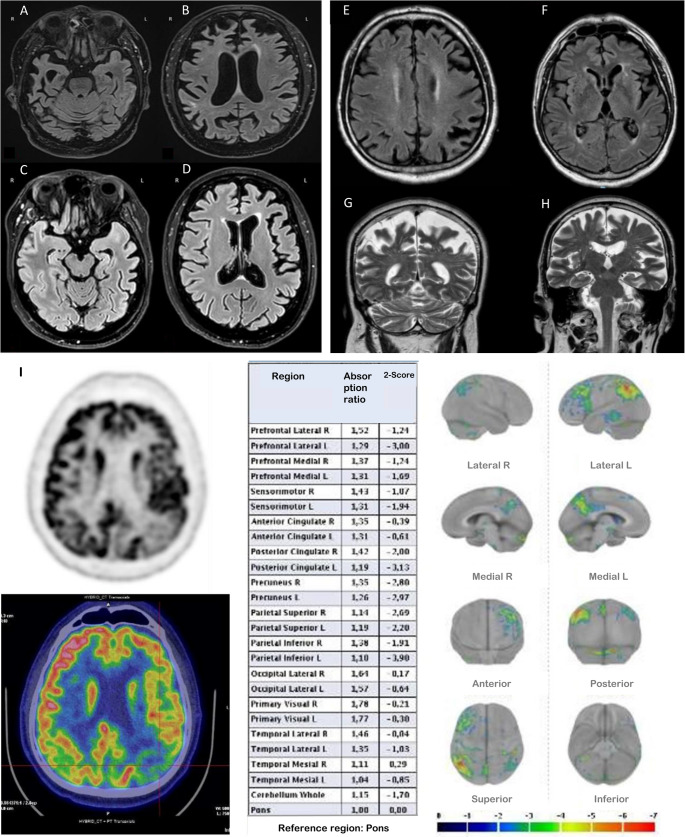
Imaging results. A, B Patient 1, proband: MRI FLAIR sequences show prominent right frontotemporal atrophy. C, D Patient 2, sister: MRI FLAIR sequences show slight left temporal atrophy and enlargement of left ventricle. E-H Patient 3: MRI axial FLAIR (E, F) and coronal T2-weighted sequences (G, H) show progression of cortical atrophy involving insula, frontal and bilateral parietal regions, more evident on the left. (I) Patient 3: Brain FDG-PET with qualitative evaluation and confirmation through statistical parametric evaluation, performed using CORTEX ID, showing hypometabolism in the left inferior parietal region and in the left lateral prefrontal cortex, posterior cingulate, and precuneus
